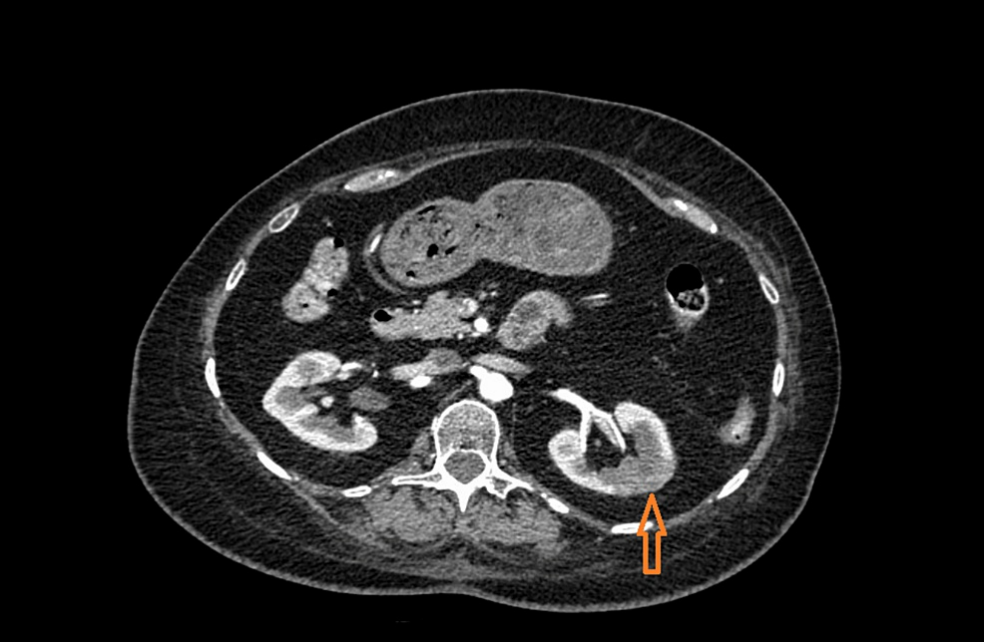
CT of the abdomen and pelvis with intravenous contrast The orange arrow shows left renal infarction.
